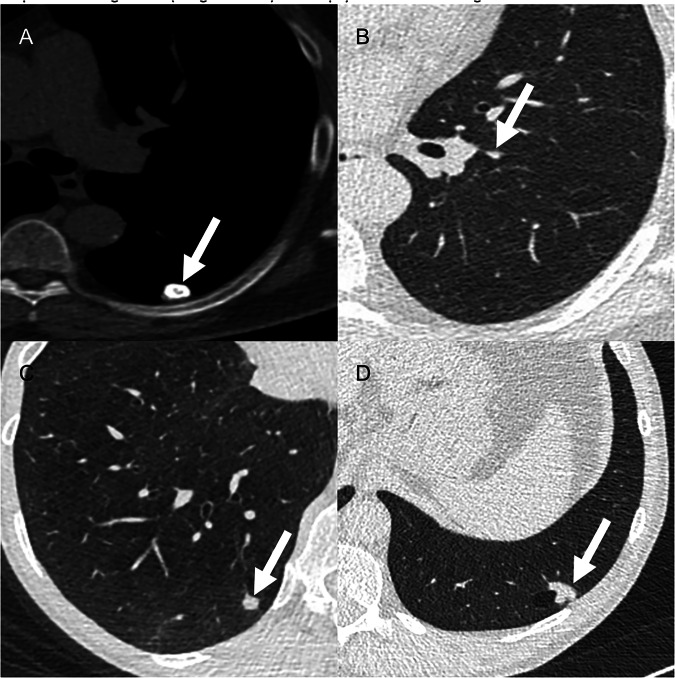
A Axial low-dose CT shows a solid nodule (arrow) in the left lower lobe with peripheral calcifications, consistent with pulmonary hamartoma (Lung-RADS 1). B Axial LDCT shows a perifissural solid nodule (arrow) in the left lower lobe, triangular in shape and measuring < 10 mm in the maximum diameter, likely representing an intrapulmonary lymph node (Lung-RADS 2). C Axial low-dose CT reveals a solid nodule (arrow) in the right lower lobe with a maximum diameter of 7 mm, categorized as Lung-RADS 3. D Axial low-dose CT shows a solid nodule (arrow) associated with a thin-walled cyst, measuring 18 mm in maximum diameter. Morphological features raised suspicion for lung cancer (Lung-RADS 4B), and biopsy confirmed the diagnosis of an adenocarcinoma
